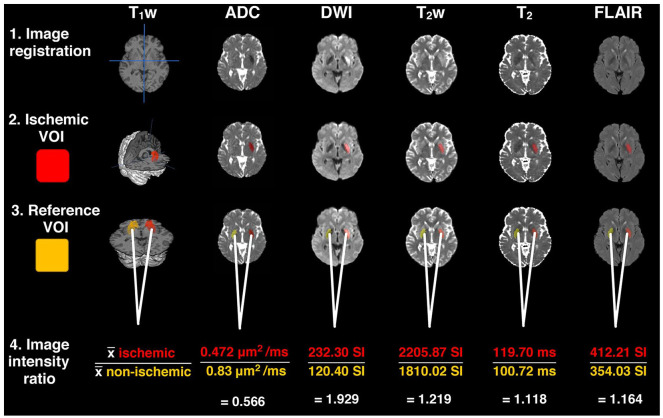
Summary of image processing and steps. (1) All images were registered to the same space. (2) ADC and T_2_ limits defined the ischemic VOI. (3) Nonischemic reference VOIs were created by reflecting the ischemic VOI across the vertical axis, applying ADC and T_2_ limits and manually editing if necessary. (4) Image intensity ratios were determined by dividing the average image intensity of the ischemic VOI by the average image intensity in the nonischemic VOI. Images shown are from the same patient (time from onset = 6 h 49 min, age = 59, thrombolysed before MRI, NIHSS = 13) and are representative of the image quality of scans acquired for all patients. ADC indicates apparent diffusion coefficient; VOI, volumes of interest.
